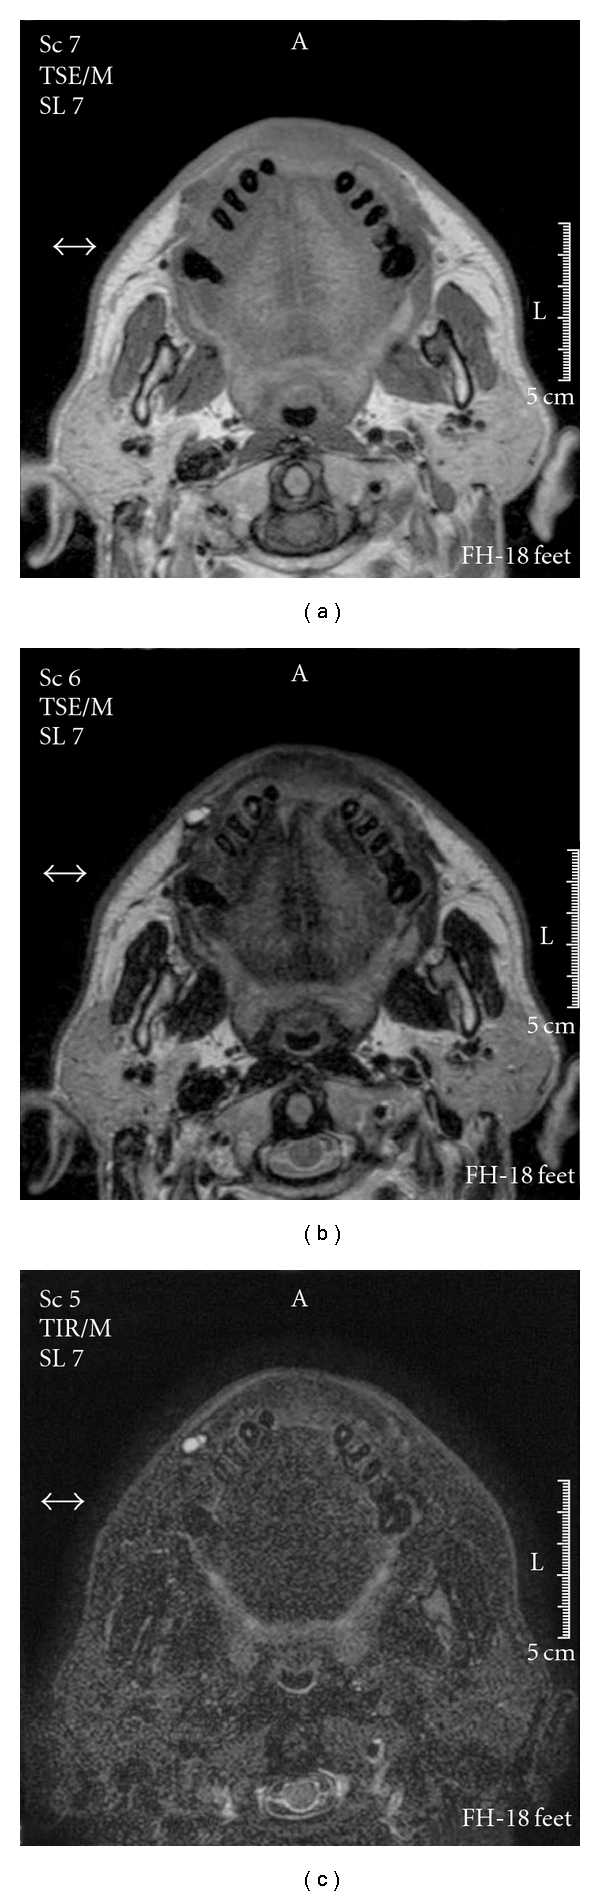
MR imaging of Case 2. (a) T1-weighted image, (b) T2-weighted image, and (c) short T1 inversion recovery image.
